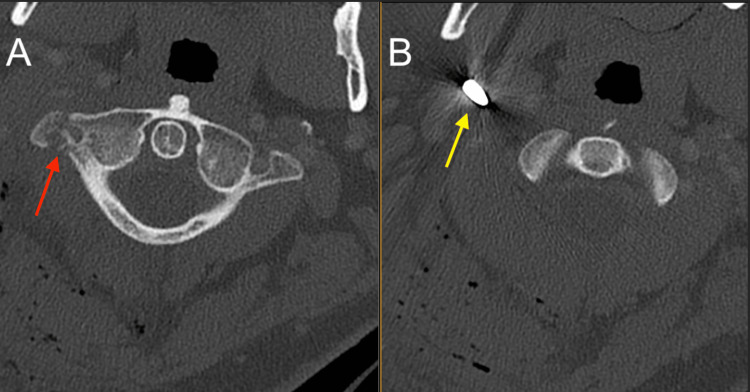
A CT scan of the cervical spine. (A) C1 right transverse process fracture (red); (B) Metallic foreign body (yellow) CT: computed tomography
